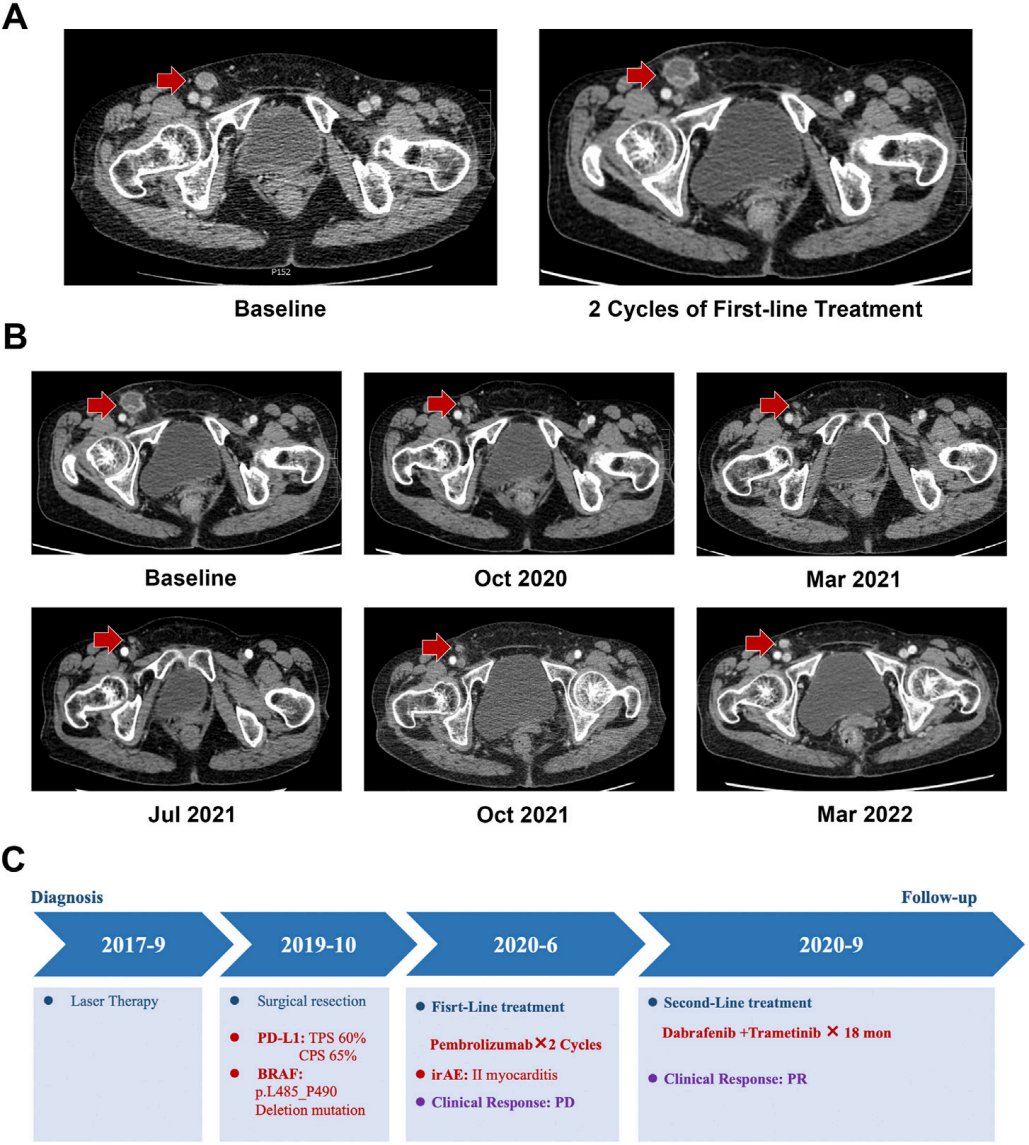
Medical imaging examination during the treatment period. (A) Progression disease (PD) confirmed by CT after two cycles of the first-line treatment. (B) Contrast-enhanced abdominal CT showed that the target lesion presented partial response (PR) to the dabrafenib and trametinib combination treatment. (C) Treatment summary of the metastatic melanoma patient with BRAF *p*.L485–P490 deletion mutations.
